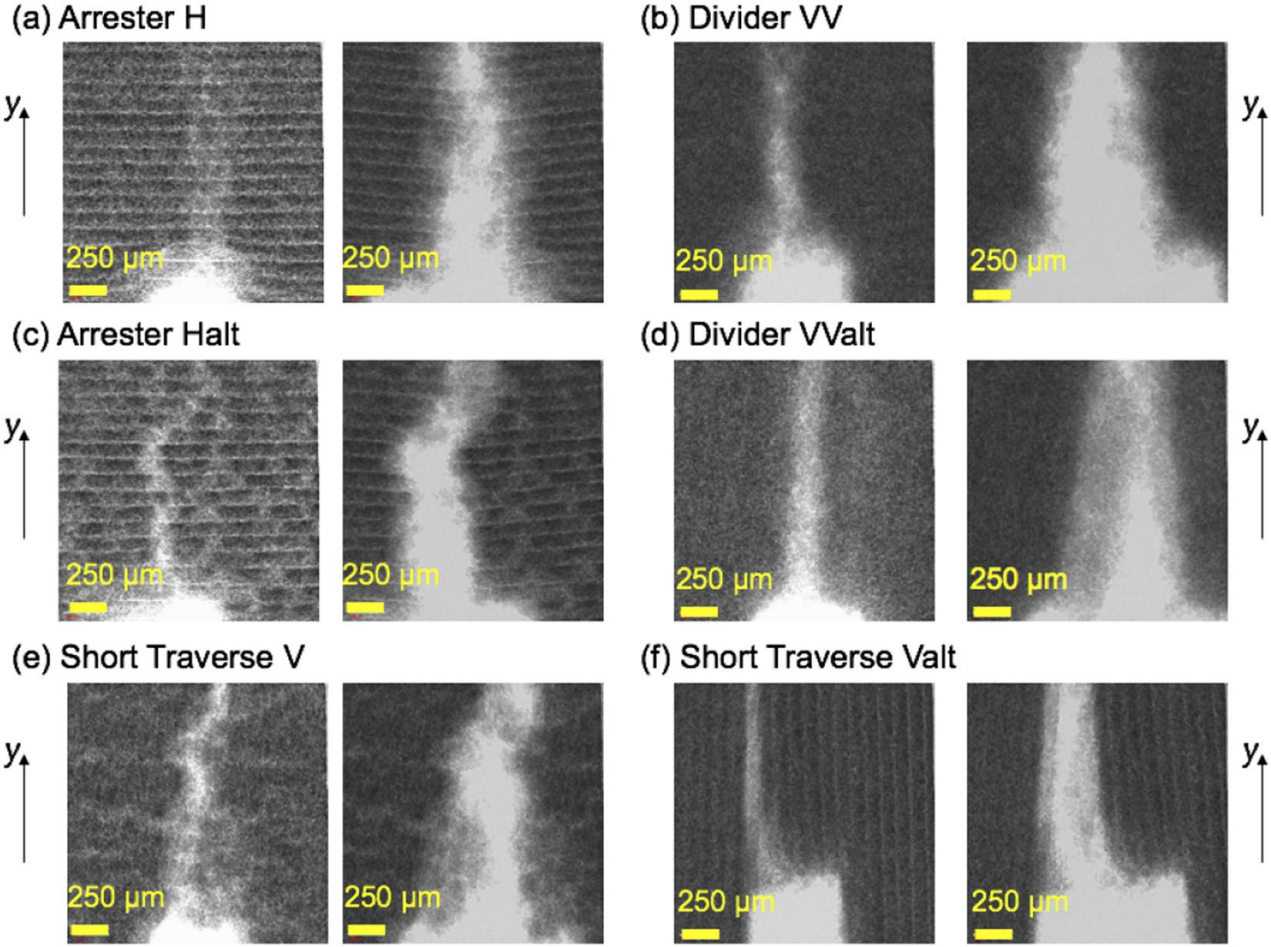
2D X-ray radiographs of the small geo-architected samples at 5% of peak load (left) and just prior to complete failure (right). The direction of fracture propagation from the notch (at the bottom of each image) is in the y-direction. The x-direction is into the page.
